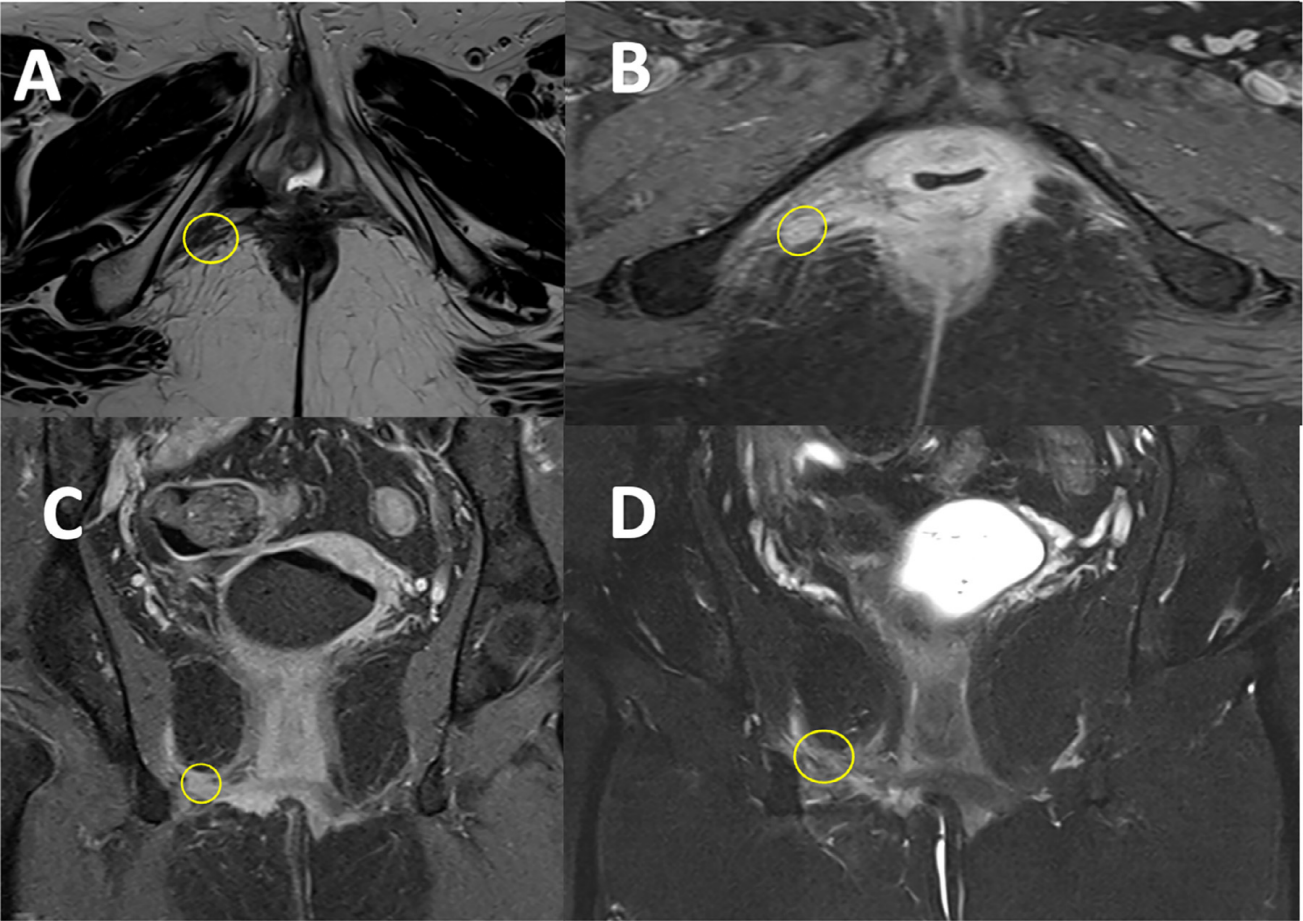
October 2023: MRI scan performed 3 months after SABR showed significant regression of the lesion, becoming isointense and no further contrast enhancement. Tumor Board review confirmed this to be nonviable fibrotic tissue (A, T2w axial; B, T1w axial with contrast; C, T1w coronal with contrast; D, T2w fat-suppressed coronal).
